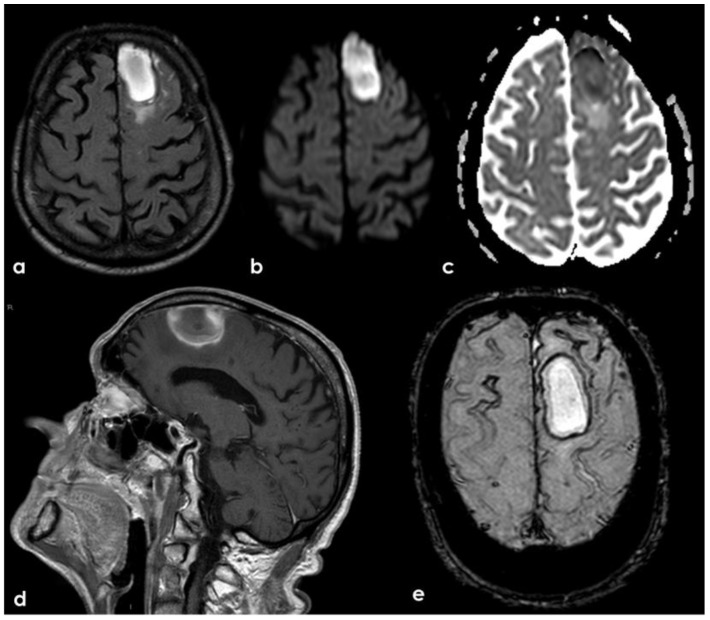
Brain MRI showing a left anterior frontal ICH on FLAIR (panel (a)), DWI (panel (b)), ADC map (panel (c)), coronal T1WI (panel (d)), and SWI (panel (e)).
